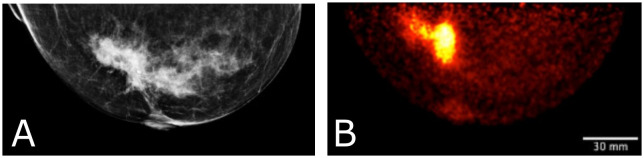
Clinical images of a patient with invasive lobular carcinoma who underwent x-ray mammography (A) and Radialis PET imaging 4-hours after radiotracer injection. The 50 year old patient (B) received 188 MBq ^18^F-FDG injection and the image shows a craniocaudal (CC) view mammography and PET image. SUV_mean, LBM_ for the lesion in (B) is 6.8, with SUV_max, LBM_ equal to 14.9.
